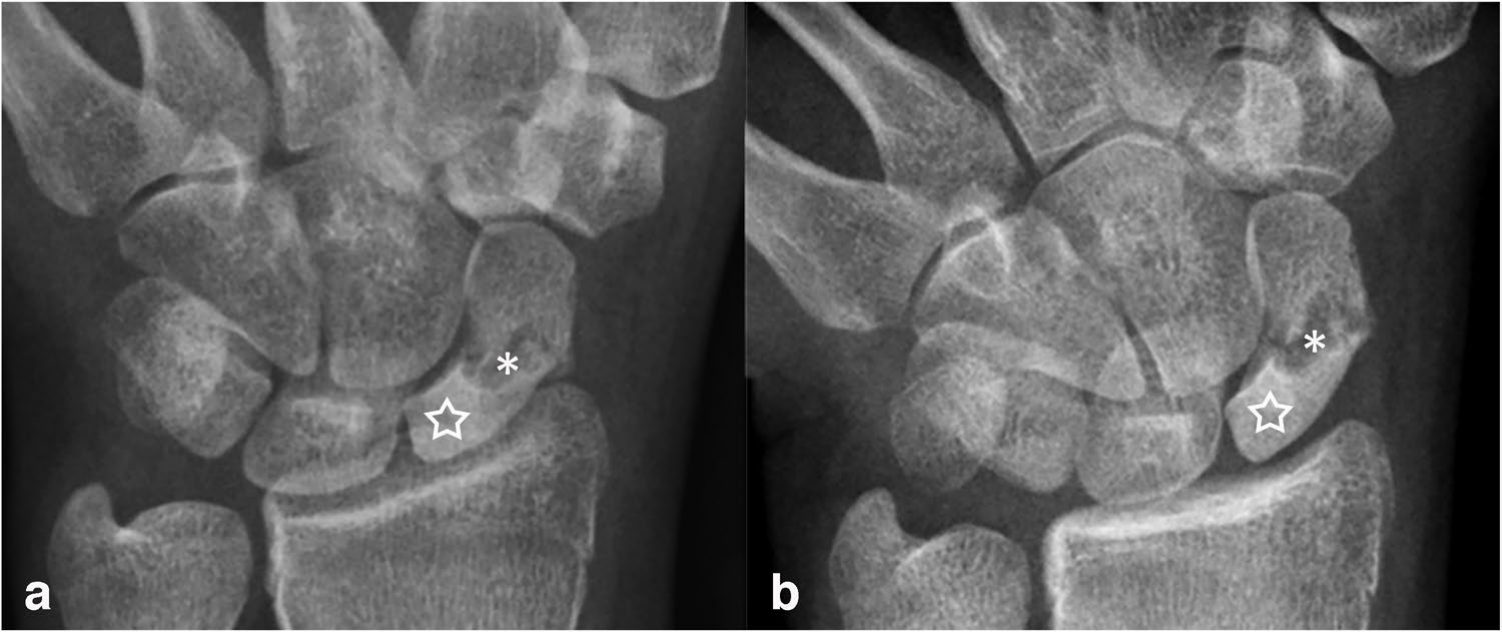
Osteonecrosis of the proximal scaphoid in a skeletally mature 15-year-old girl. PA (a) and PA scaphoid (b) radiographic views show a waist fracture with lobulated resorption (asterisks) and increased radiodensity of the proximal scaphoid (stars). The patient underwent ORIF with an iliac crest autograft
